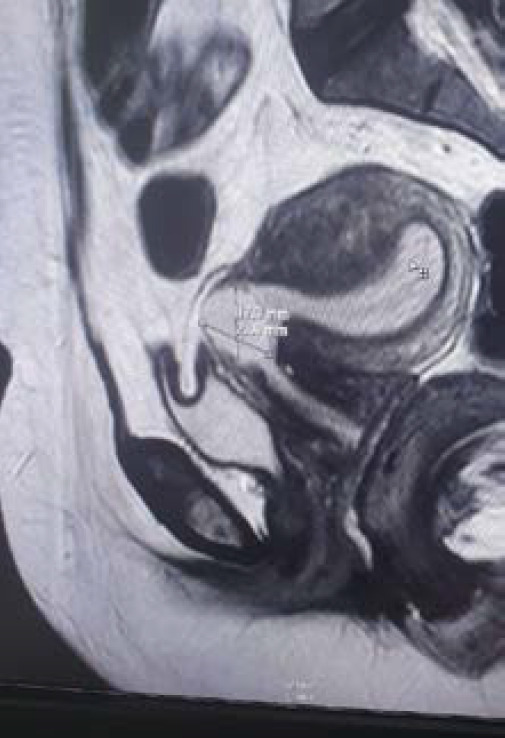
MRI showed a well-defined cystic lesion of 21 X 19mm^2^ in the anterior aspect of the lower uterine segment embedded within the previous cesarean scar.
